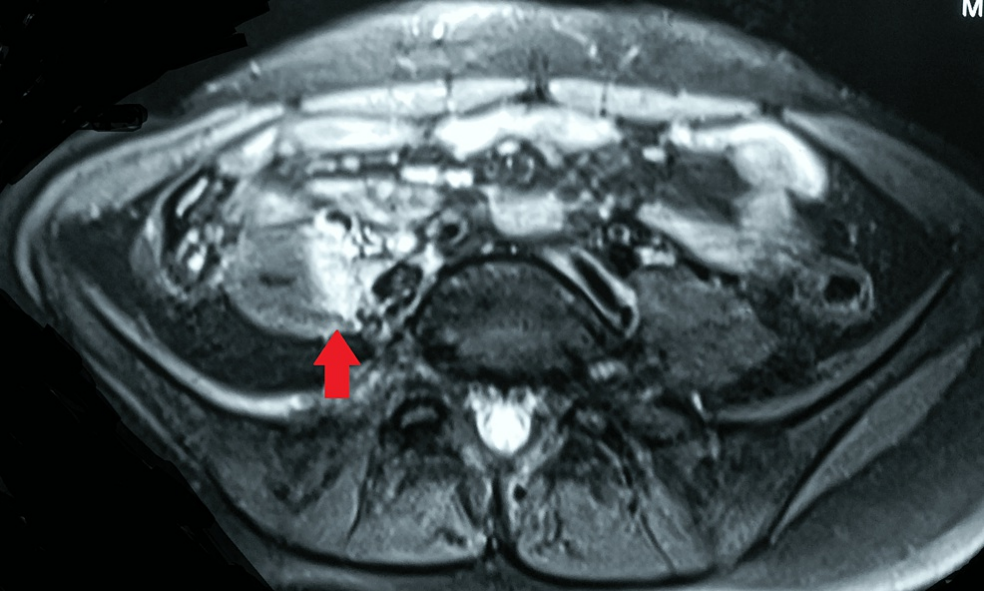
MRI showing the abscess MRI: magnetic resonance imaging
